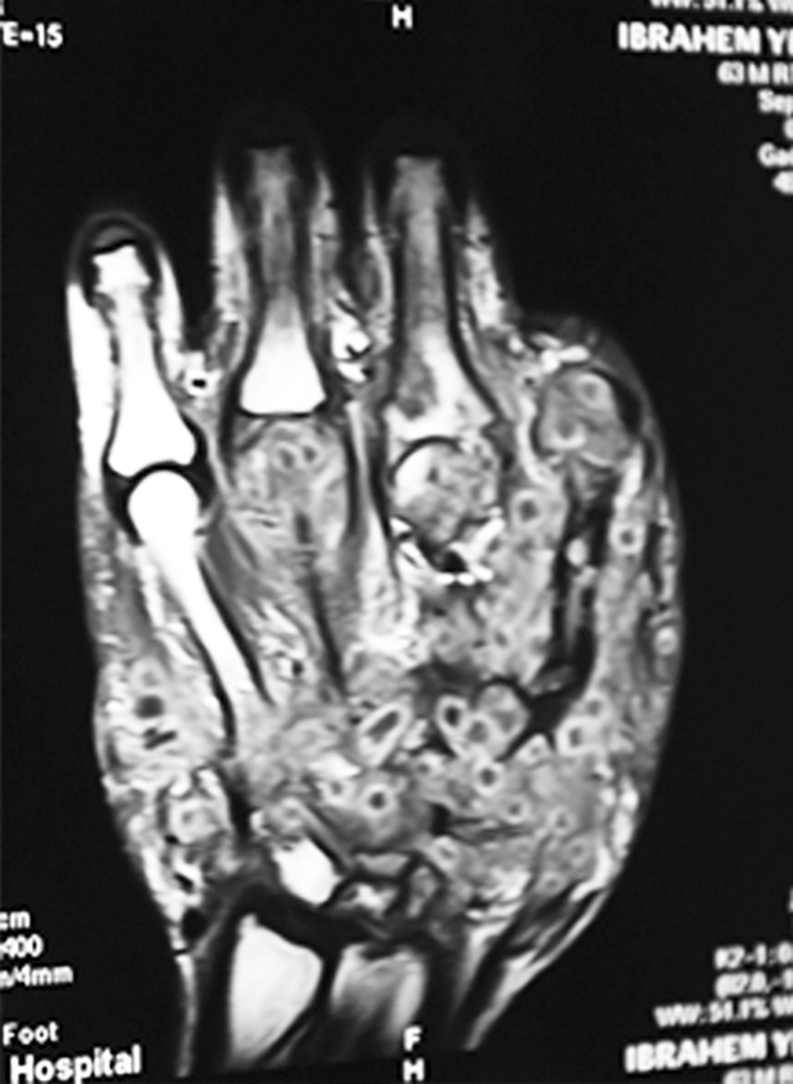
MRI showing involvement of soft tissue and bone by mycetoma and the dot-in-circle sign which is characteristic of for mycetoma.
